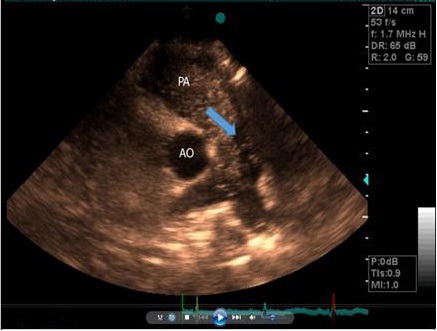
short axis parasternal view after agitated saline injection showing free space site in lateral of main PA in favor of false lumen with delay filling (Blue arrow).
